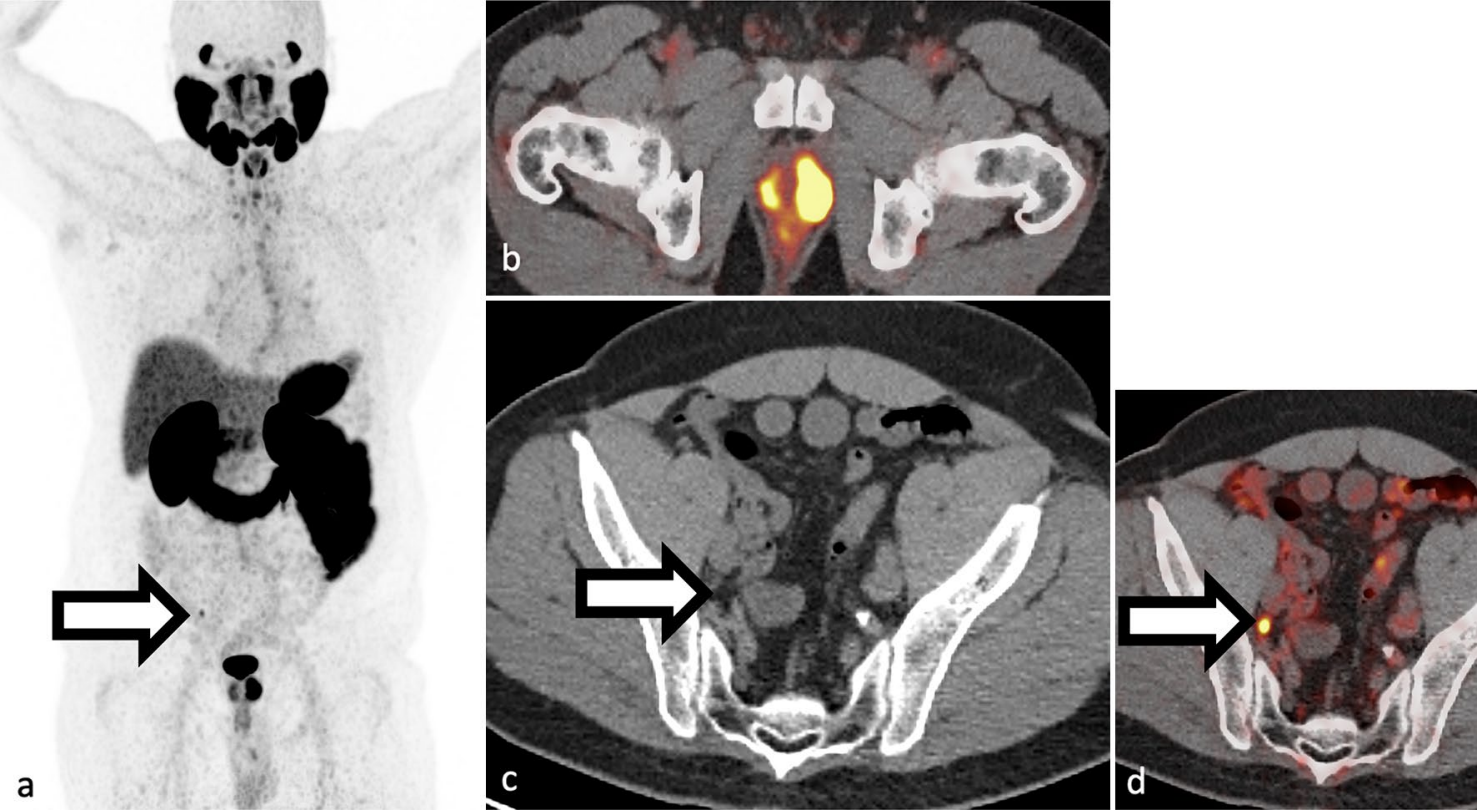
74-year-old with high-risk prostate cancer. Pre-surgical planning 68Ga-PSMA-11 PET/CT performed. Single PSMA avid 2 mm right internal iliac chain lymph node (white arrow in panels a and d; SUVmax 4.5), not well seen on CT (white arrow on panel c). Maximum intensity projection PET a is scaled at 0–5 SUV
